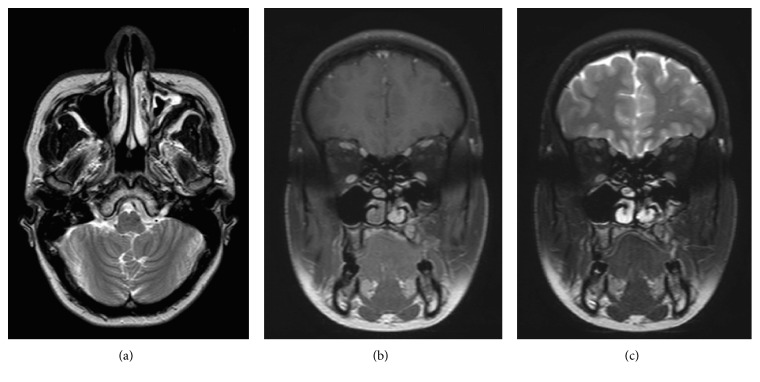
(a, b, c) Respectively, axial T2, coronal T1, and coronal T2 postoperative MRI showing mucosal thickening in the maxillary without any evidence of tumor recurrence.
